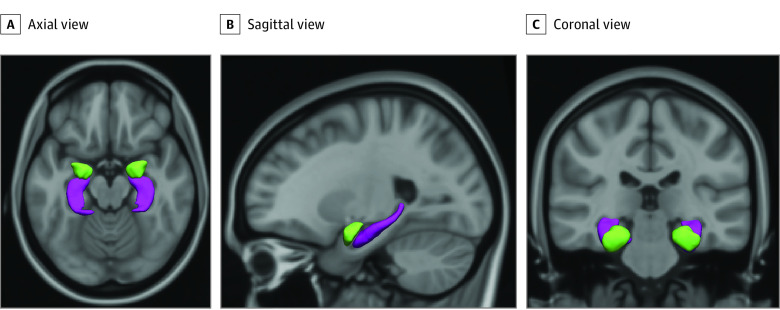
T1-Weighted Structural Magnetic Resonance Imaging (MRI) Scan (Axial, Sagittal, and Coronal View) Showing the Amygdala and Hippocampus Segmentation The amygdala is shown in green, and the hippocampus is shown in purple. Figure adapted from Cortes Hidalgo et al.^52^
